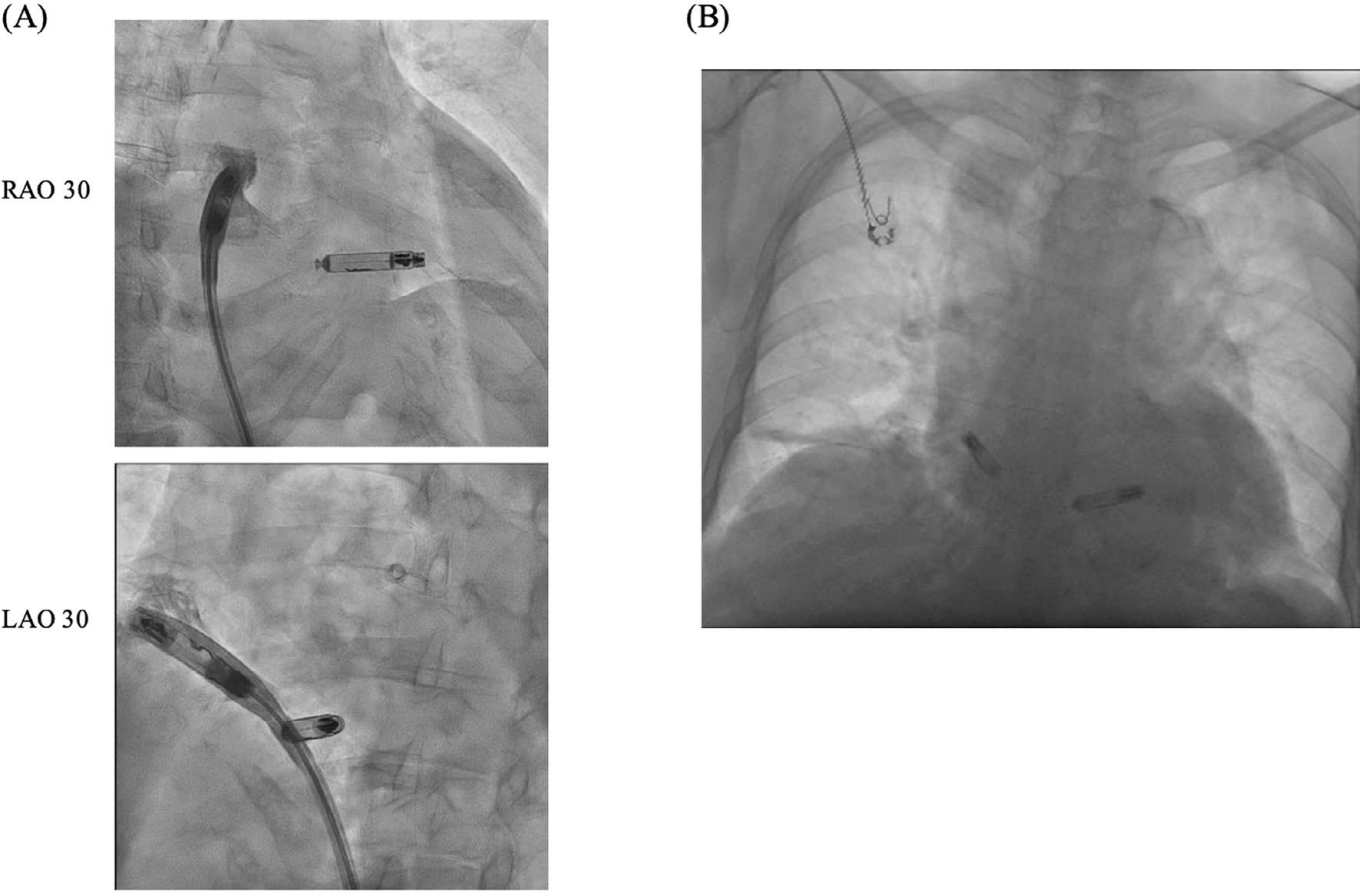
Fluoroscopic images during and after dual‐chamber leadless pacemaker implantation. (A) Fluoroscopic views during the procedure. Right anterior oblique (RAO) and left anterior oblique (LAO) projections showing the ventricular leadless pacemaker positioned in the right ventricle. Contrast injection through the atrial leadless pacemaker delivery catheter delineates the right atrial appendage. (B) Chest radiograph obtained immediately post‐implantation showing final positions of both atrial and ventricular leadless devices. The atrial device is positioned in the right atrial appendage and the ventricular device in the right ventricular.
